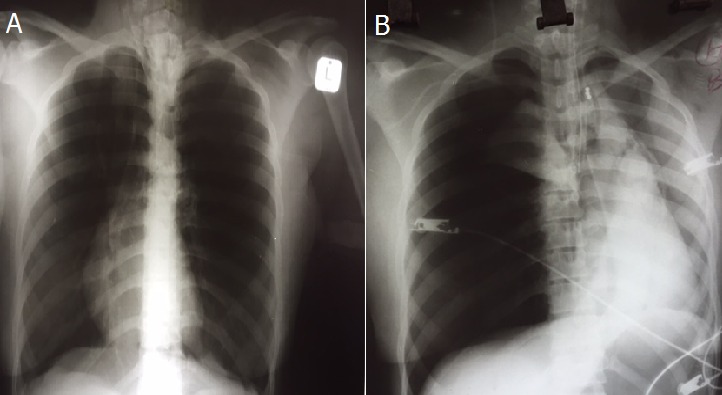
A): anterior Posterior bed side chest radiograph demonstrating dextrocardia with air trapping; B): tension pneumothorax displacing heart to left
